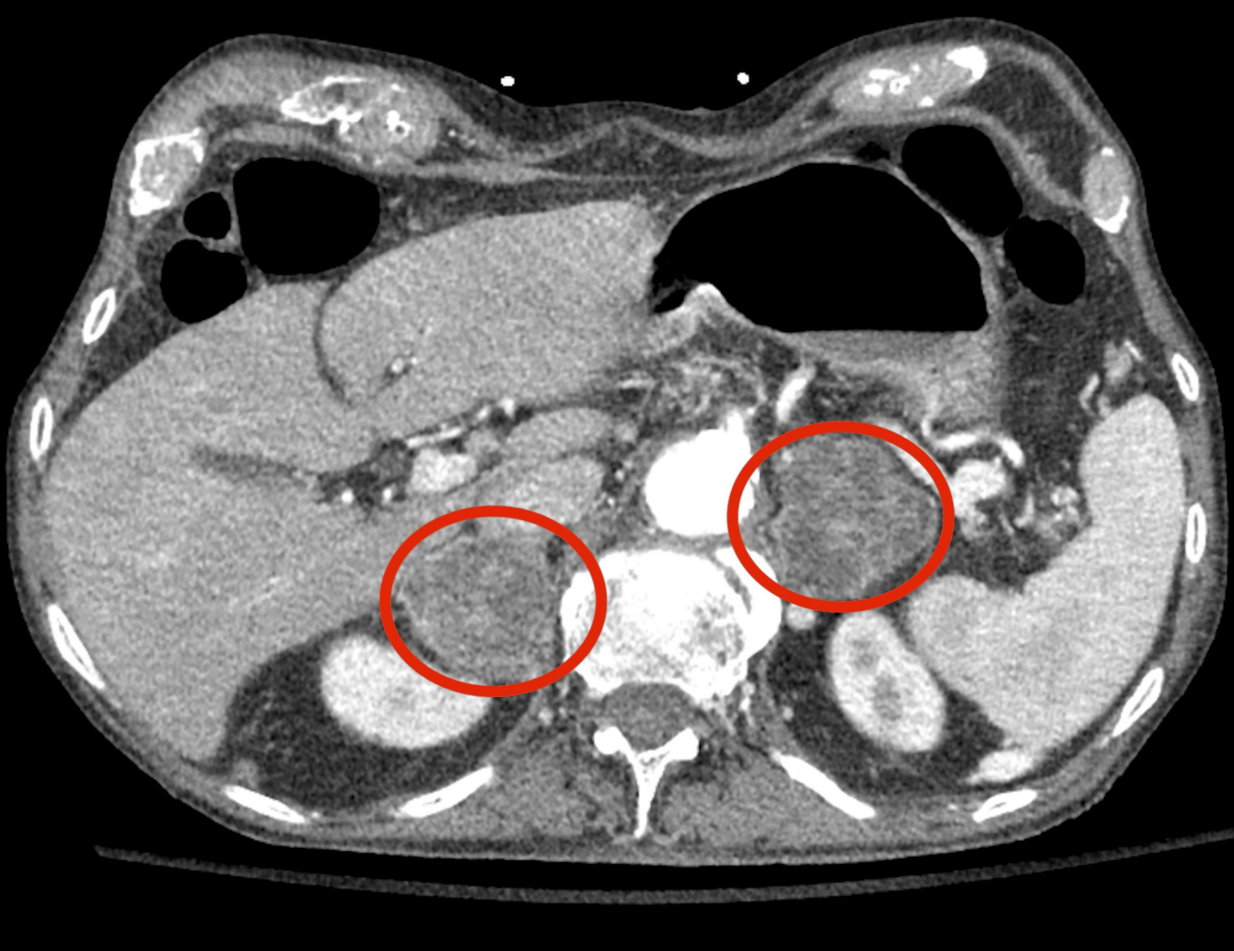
CT abdomen and pelvis with contrast demonstrating bilateral adrenal masses (red circles).
